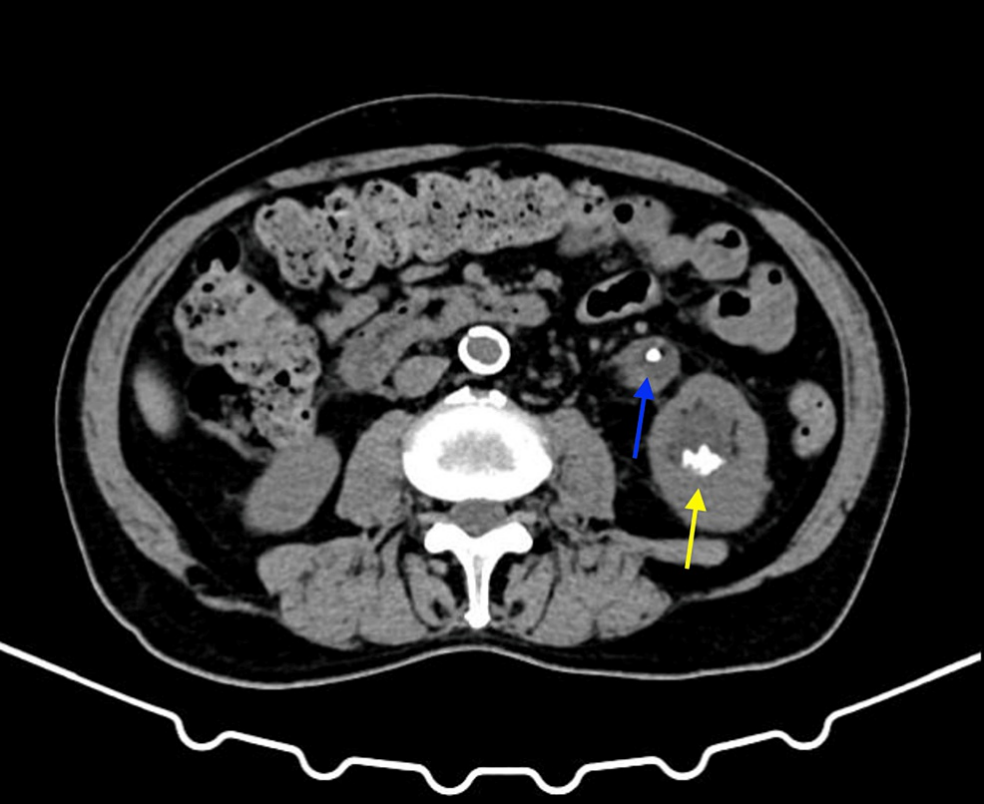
A non-contrast computed tomography for the ureter and urinary bladder (CT-KUB) Axial section showing both the left double-J stent (blue arrow) and the lower pole left renal stone (yellow arrow)
